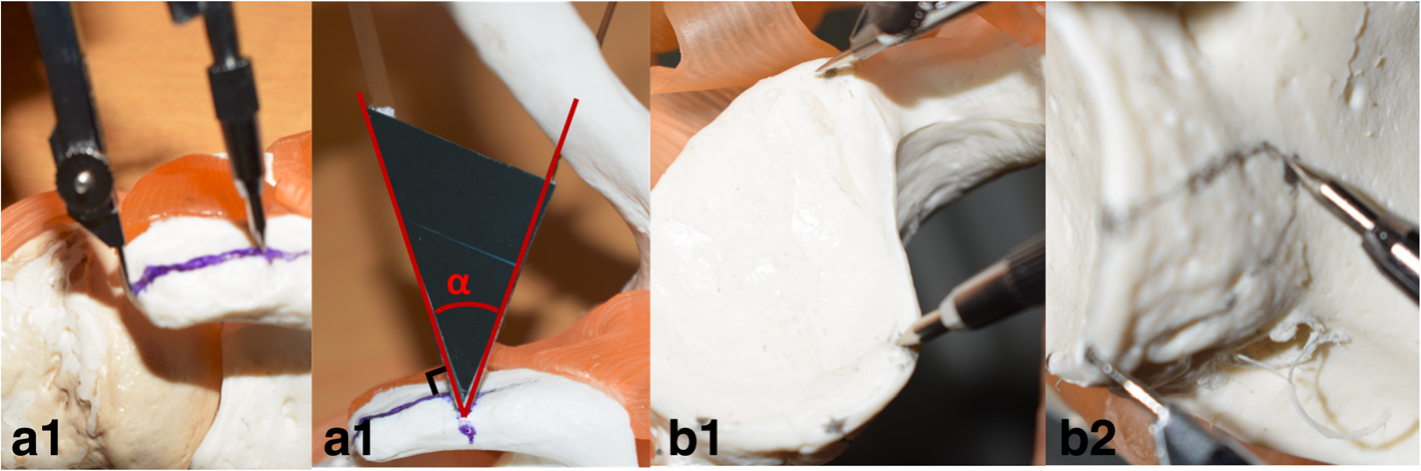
The intraoperative sketch. The calliper was for measuring length, and the X-ray film was for measuring angle. A1–A2 The coracoid osteotomy parameters. B1–B2 The glenoid bed fixation data
